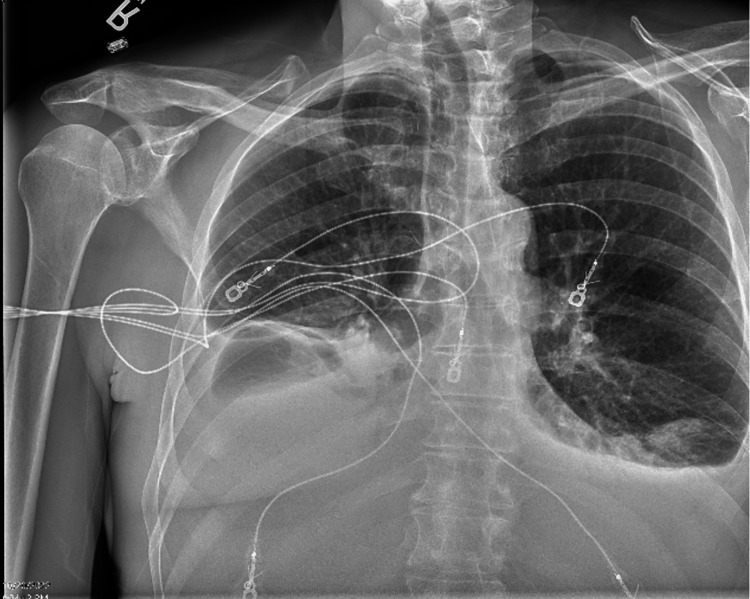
Chest X-ray with bibasilar consolidations with bilateral pleural effusions right greater than the left
